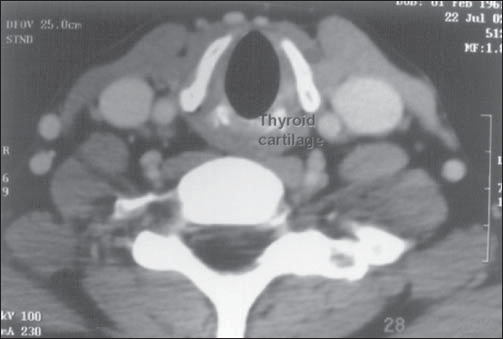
Computed tomography of the larynx, demostrating thyroid absence.
